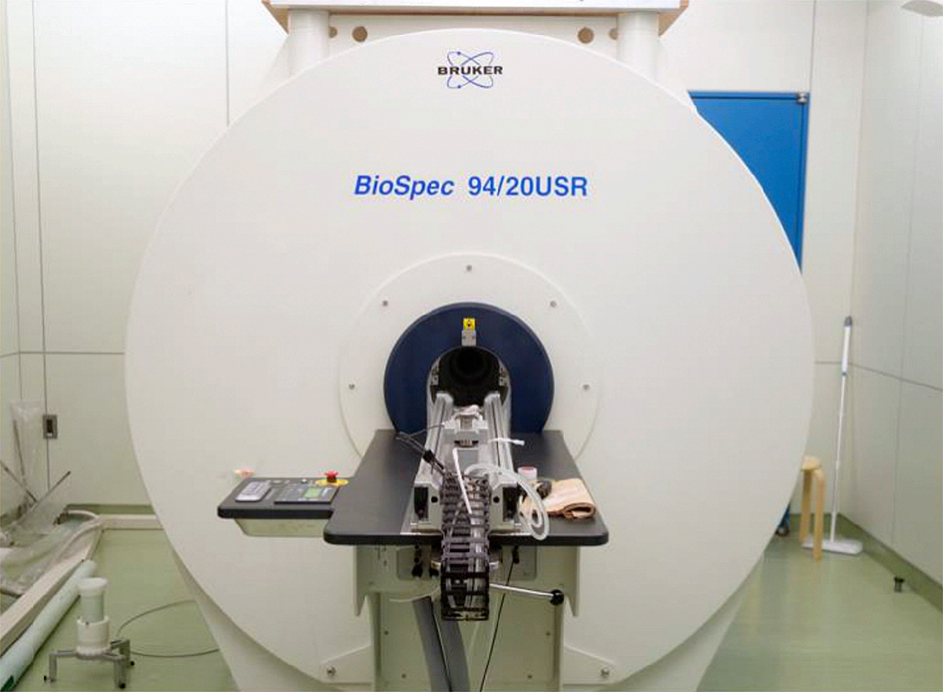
An ultra-high magnetic field magnetic resonance scanner (9.4-T) manufactured by Bruker (BioSpec 94/20 USR) was used for magnetic resonance imaging. The sequence was as follows: repetition time 3,500 msec; Echo time 11.0 msec; Flip angle, 180°.
